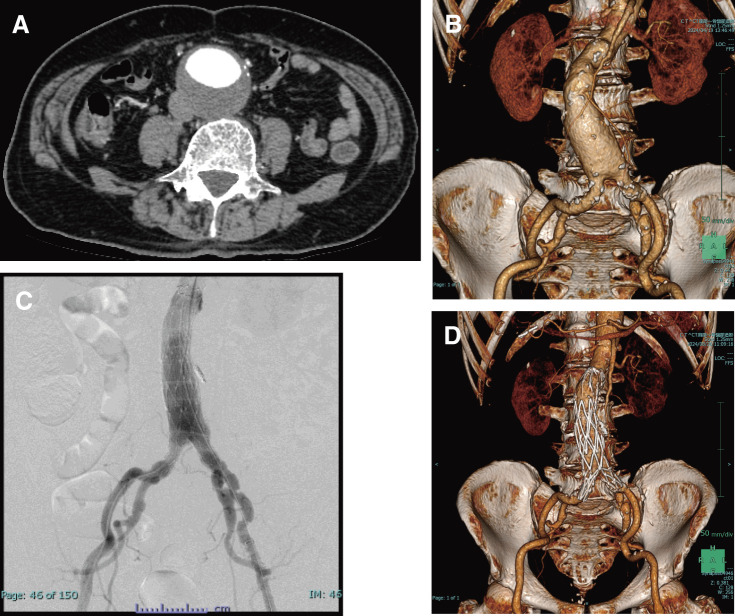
Fig. 1 (A) Preoperative enhanced CT showed a 51-mm AAA. (B) Preoperative three-dimensional CT. (C) The post-EVAR angiogram of the abdominal aortic artery demonstrated preservation of both internal iliac arteries with no endoleak. (D) Postoperative CT showed exclusion of the aneurysm preservation of both renal arteries as well as both internal iliac arteries with no endoleak. CT: computed tomography; AAA: abdominal aortic aneurysm; EVAR: endovascular aortic repair
